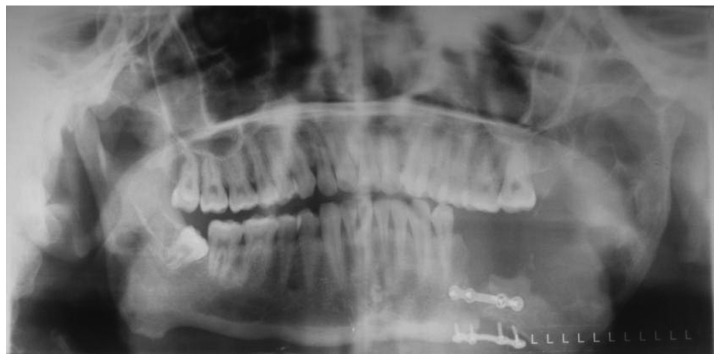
Panoramic radiograph showing an extensive, ill-defined, multilocular radiolucent lesion prior to the second surgery.
